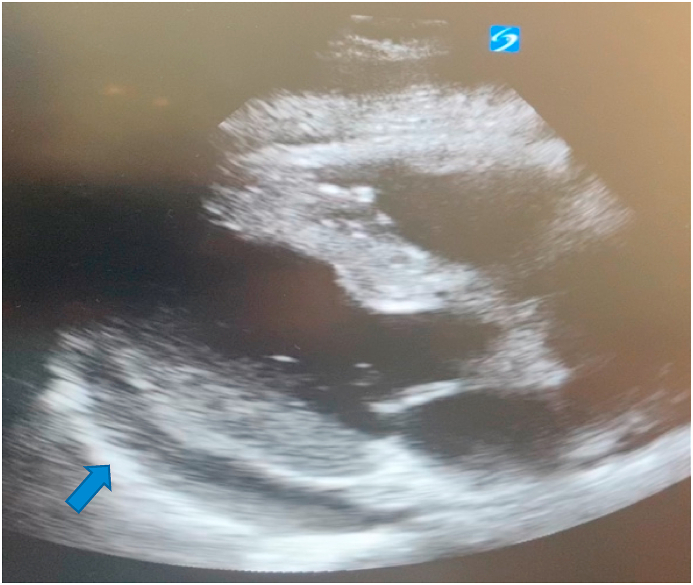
Parasternal long axis view, showing pericardial effusion.
